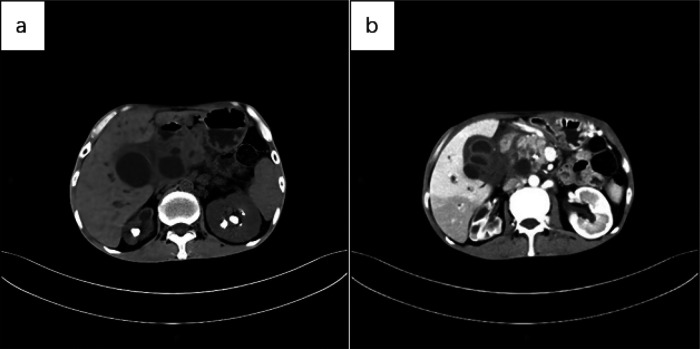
(a) CT scan of the abdomen showed multiple nodular high-density shadows in both kidneys, with significant reduction in the volume of the right kidney and uneven thickening of the renal cortex, suggestive of renal self-resection. The left kidney exhibited compensatory enlargement. Additionally, multiple cystic mass shadows were observed in the upper abdomen. (b) Abdominal CT (cortical phase) revealed uneven enhancement of the right kidney, with multiple low-density areas and increased volume of the left kidney. No significant enhancement was observed in the sac of the upper abdominal lesion, and the cystic wall showed uniform enhancement.
